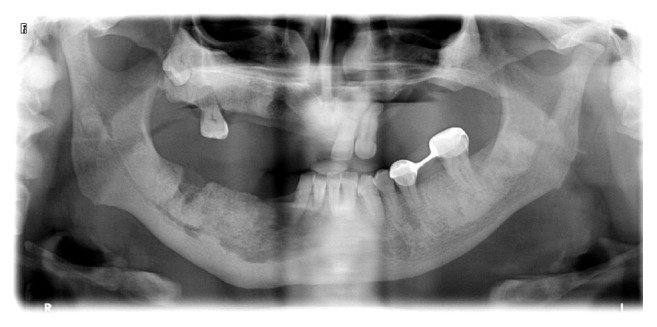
Panoramic radiography of the patient before extraction of the left maxillary central and lateral incisor in 2011.
